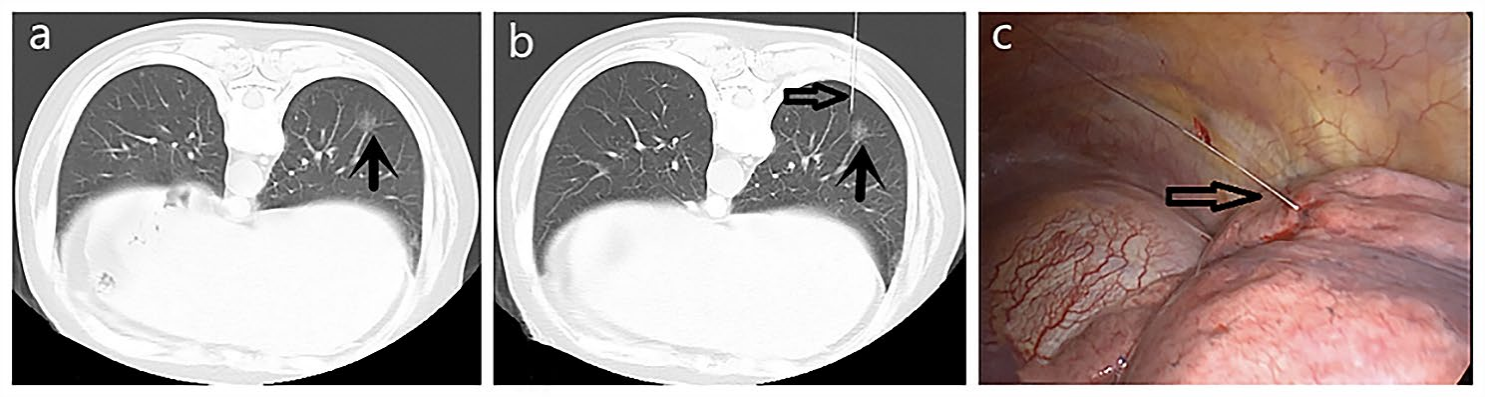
A 65-year-old female with a GGN in the right lower lobe who underwent CT-guided localization with a hook wire needle. (a) The lesion (black arrow) is observed on an axial CT image. (b) A hook wire needle (black arrow) is inserted around the lesion; postlocalization CT shows the relationship among the needle (black arrow), lesion (white arrow) and chest wall. (c) The end of the four-hook needle (white hollow arrow) is visualized during VATS.
